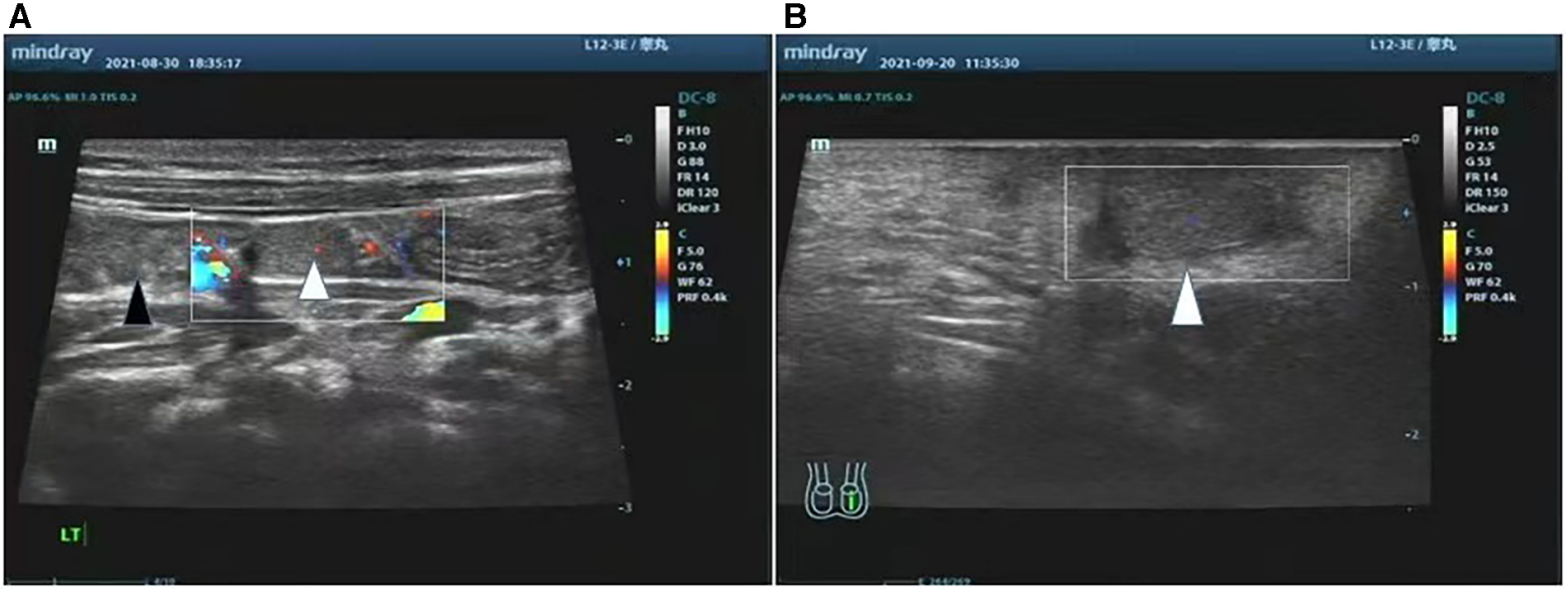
Pre-operative ultrasound and post-operative ultrasound of case 2 US examination of the left testis, it indicated the left testis was adjacent to the lower pole of the spleen (A). 1 month after surgery, the left testis was well positioned and survived (B). (Black triangle: spleen, white triangle: left testis).
